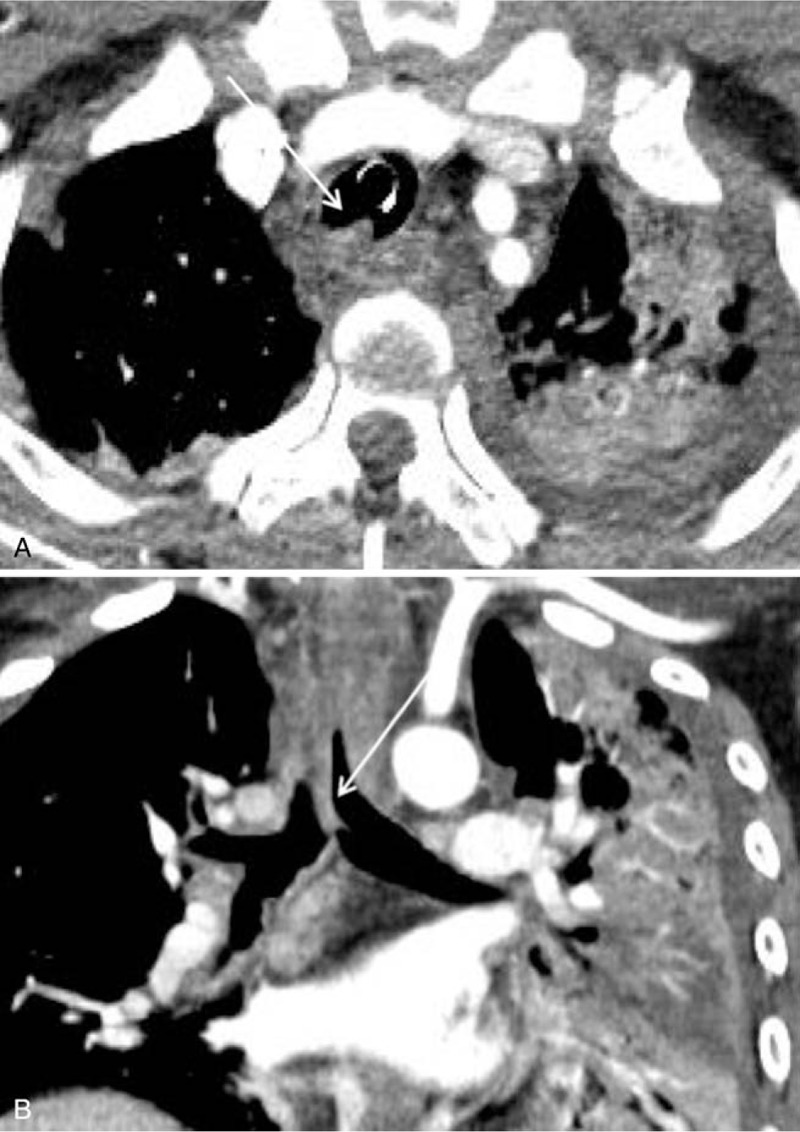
Chest CT findings of acute airway injury 5 days post ingestion. A, B. Interval-developed endobronchial lesions from the trachea to the left main bronchus with tenting shape suggest mucosal injury with swelling.
